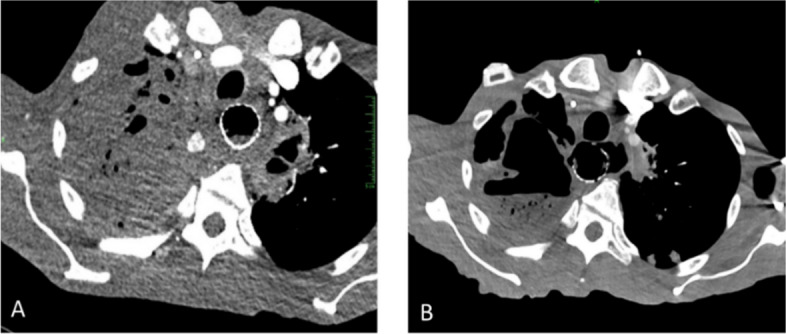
A Initial chest CTA during the first admission showing a hyperdensity measuring 1.3 cm at the medial aspect of the right upper lobe mass. B Post intervention chest CTA during the second admission showing no evidence of pseudoaneurysm
